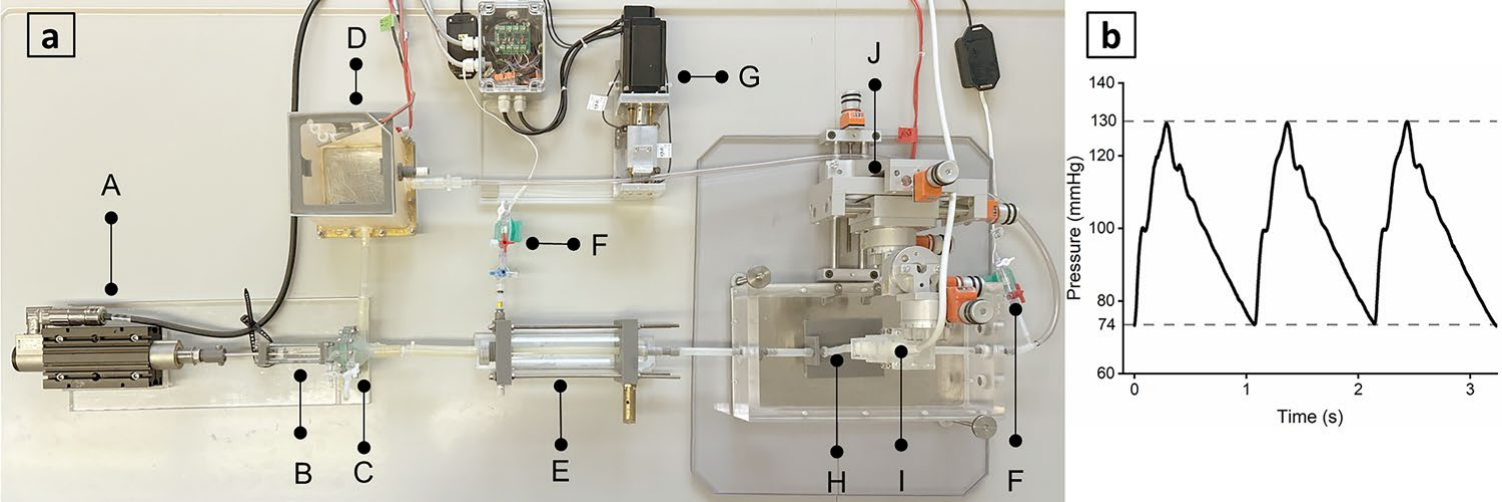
Pulsatile pump set-up (a): linear motor (A), syringe (B), pump chamber (C), reservoir (D), compliance chamber (E), pressure transducers (F), resistance (G), vessel model (H), ultrasound probe (I), ultrasound probe stabilizer (J); Pulse pressure (74–130 mmHg) waveform measured at the outlet of PVA-H model (b).
